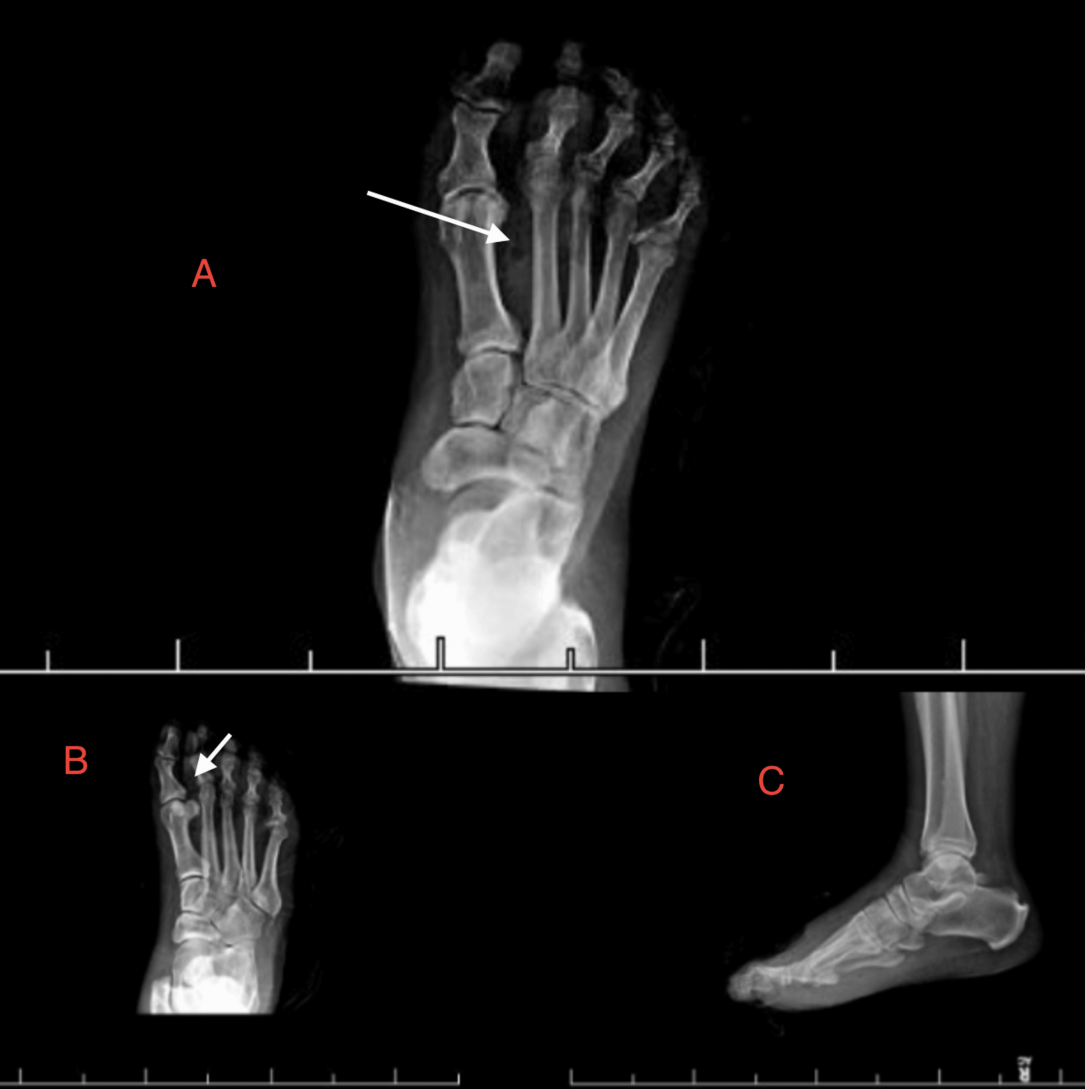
Initial right foot radiographs (A) Anteroposterior and (B) oblique views showing subcutaneous emphysema in the soft tissues (arrows), concerning for gas gangrene. Lateral view (C) provided for completeness, where the emphysema is less conspicuous. No definite radiographic evidence of osteomyelitis is seen.
